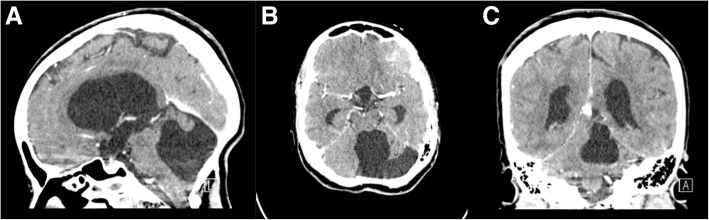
Follow-up at 20 years of age a sagittal, b axial, and c coronal CT scan demonstrating no tumor recurrence
